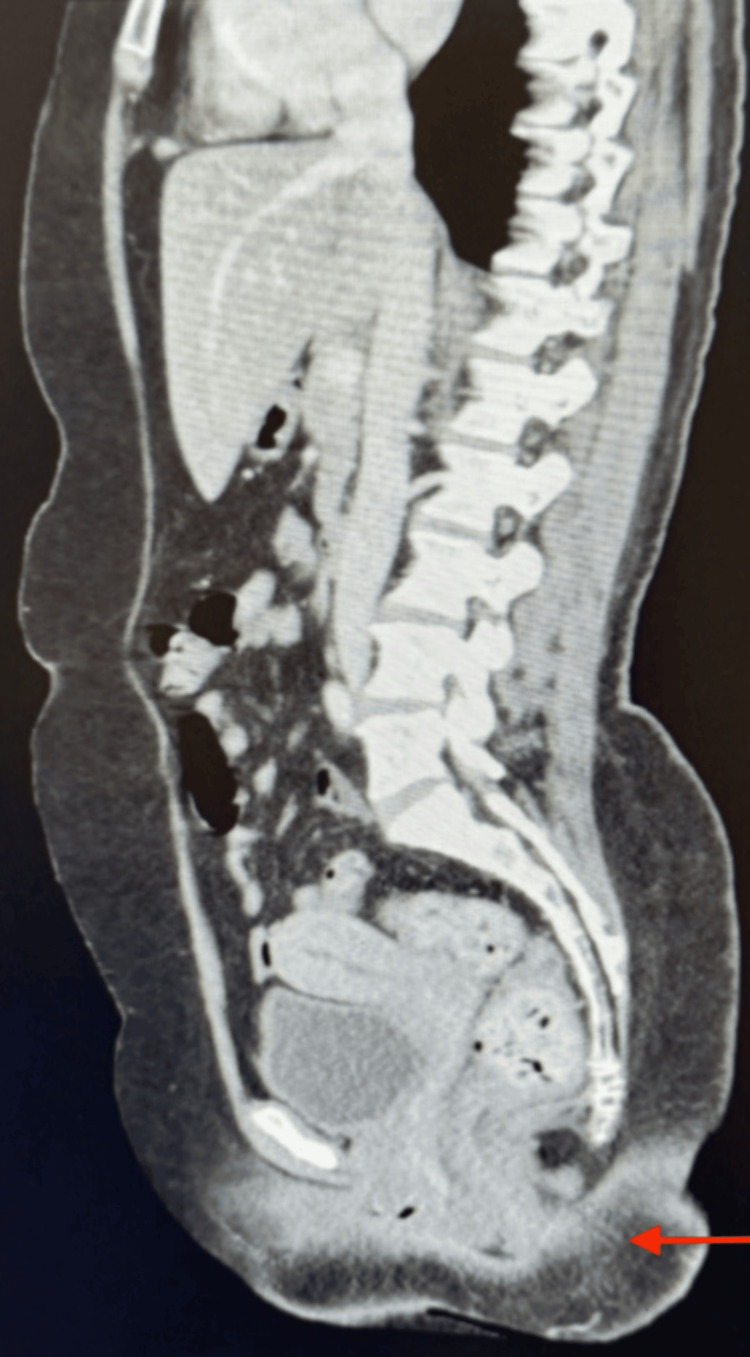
Pre-surgical enhanced CT scan of the abdomen and pelvis with IV contrast shows no abdominopelvic metastasis (arrow).
